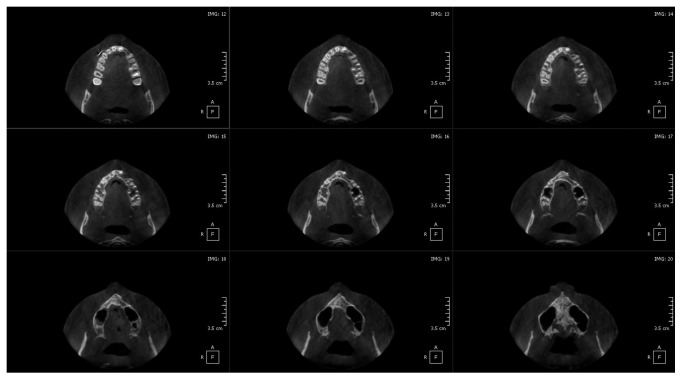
Axial view of cone beam computerized tomography showing the healing at the surgical site with the reduction of the bone width two years after surgery.
